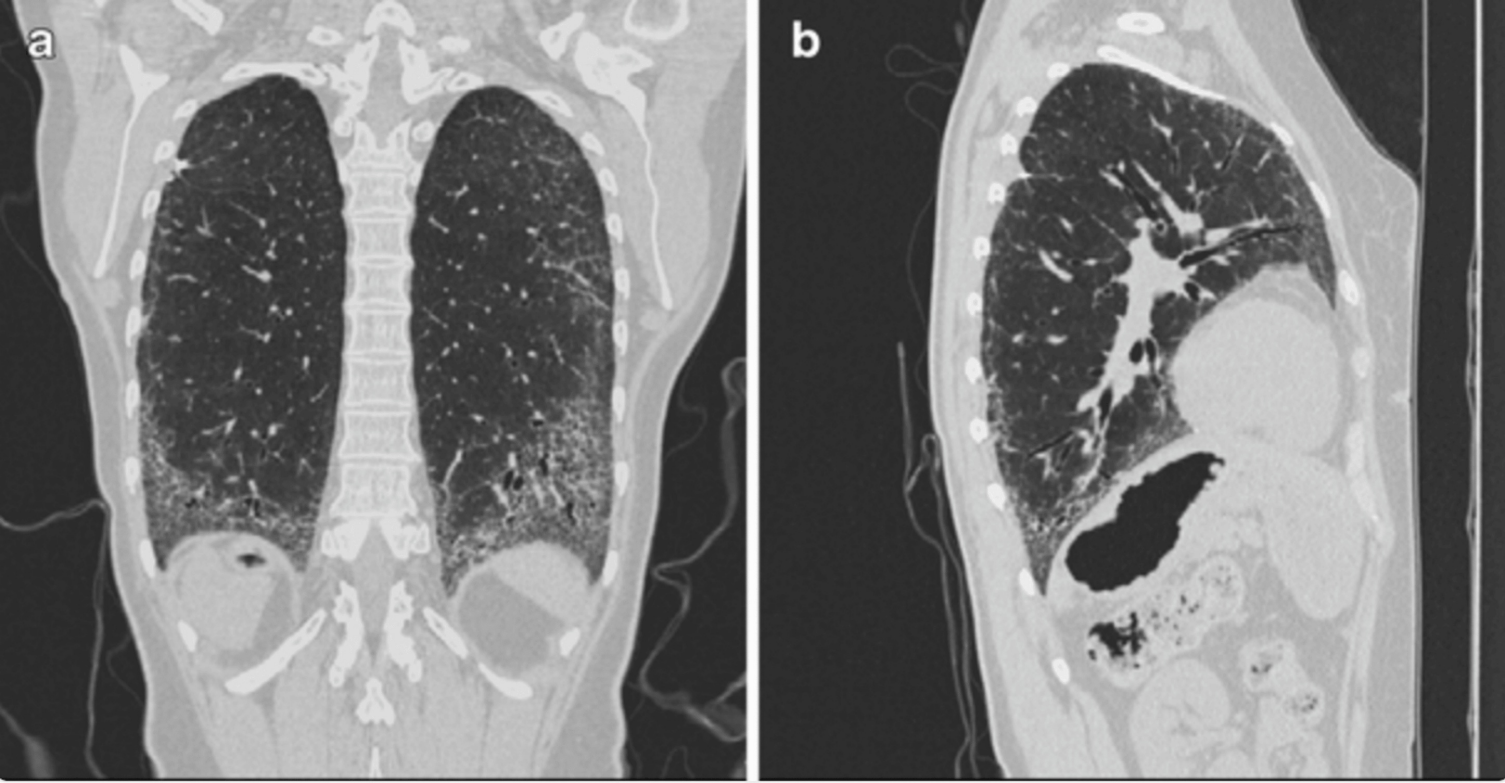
Advanced radiological features of SSc-ILD on chest CT. Coronal and sagittal chest CT reconstructions (lung window) demonstrate the classic appearance of advanced systemic sclerosis-associated interstitial lung disease (SSc-ILD) in patients with progressive disease. Findings include extensive architectural distortion due to progressive pulmonary fibrosis (a and b). Reproduced from Strollo and Goldin (2010) [10]. Image reproduced from an open-access article distributed under the terms of the Creative Commons Attribution Noncommercial License.
